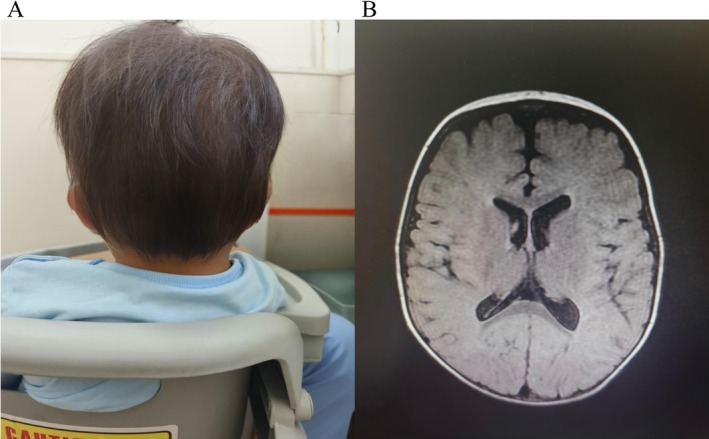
Analysis of facial features and MRI characteristics in the XLID93 proband. (A) Clinical photograph of the patient showing short neck and low posterior hairline. (B) MRI scans of the proband's brain reveal a few patchy slightly hyperintense signals on T2Flair around the anterior and posterior horns of the bilateral ventricles, and the bilateral ventricles were slightly widened.
